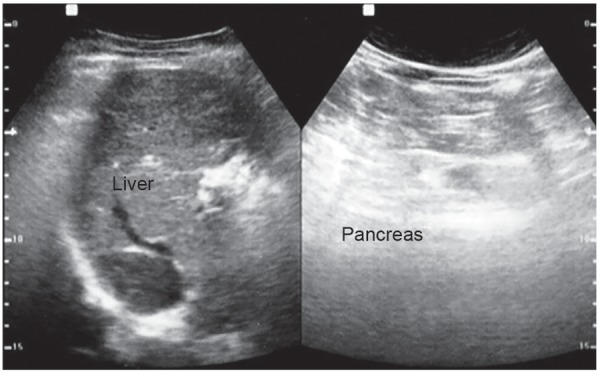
Ultrasonography of hepato-biliray system (HBS)
